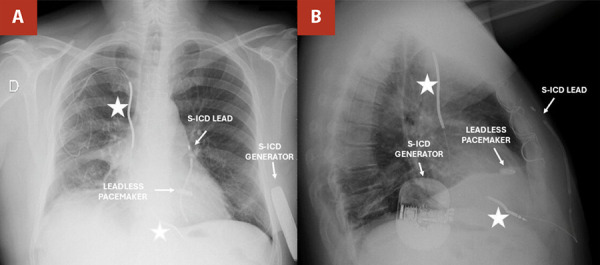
One-month post-discharge evaluation. (A, B, arrows) Posteroanterior and lateral chest X-ray: S-ICD generator located at the level of the fifth intercostal space (ICS), with the lead position at the 3rd left parasternal ICS; leadless pacemaker visible at the mid-septal region of the right ventricle (RV).
